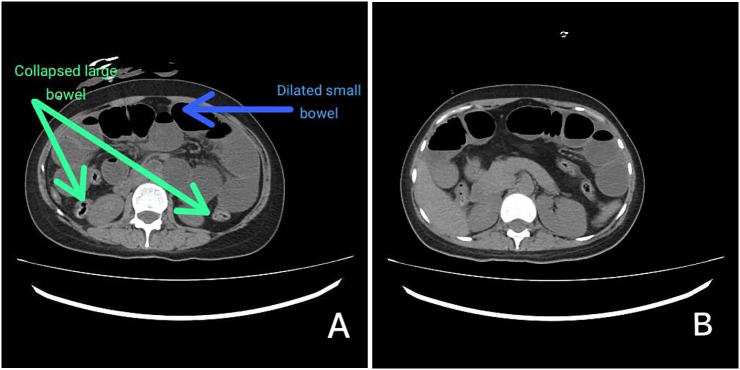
A and B. Non-contrast CT scans showing dilated bowel loops and collapsed large bowel.
